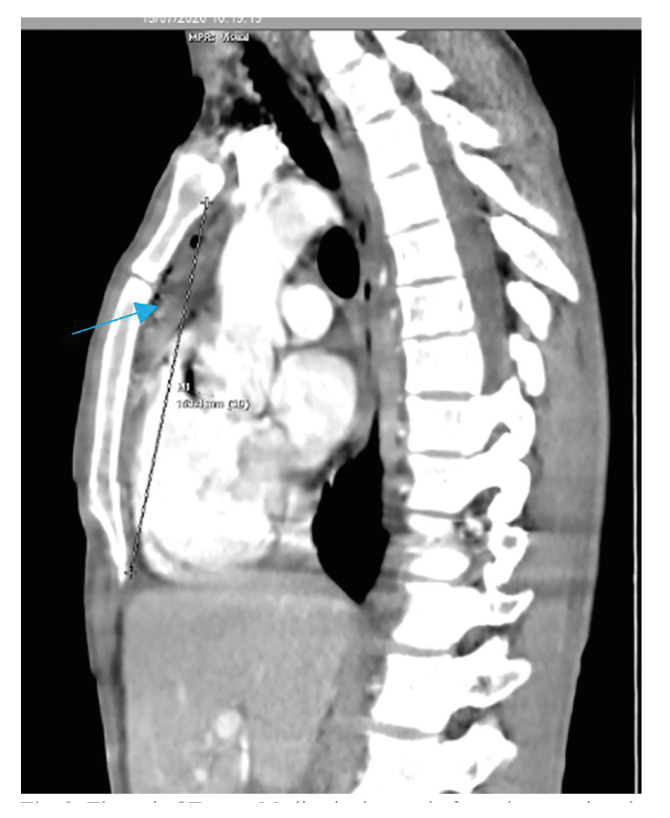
Thoracic CT scan. Mediastinal spread of an odontogenic collection (Blue Arrow).
